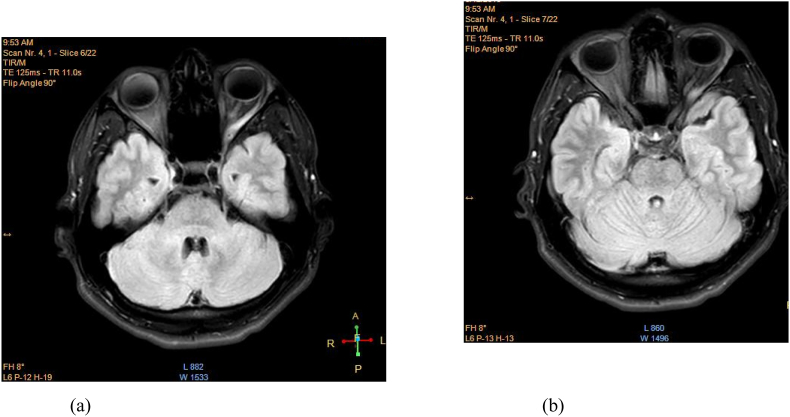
Axial section of MRI brain and orbit showing cystic lesion with myositis of left lateral rectus muscle compressing the optic nerve at the orbital apex.
